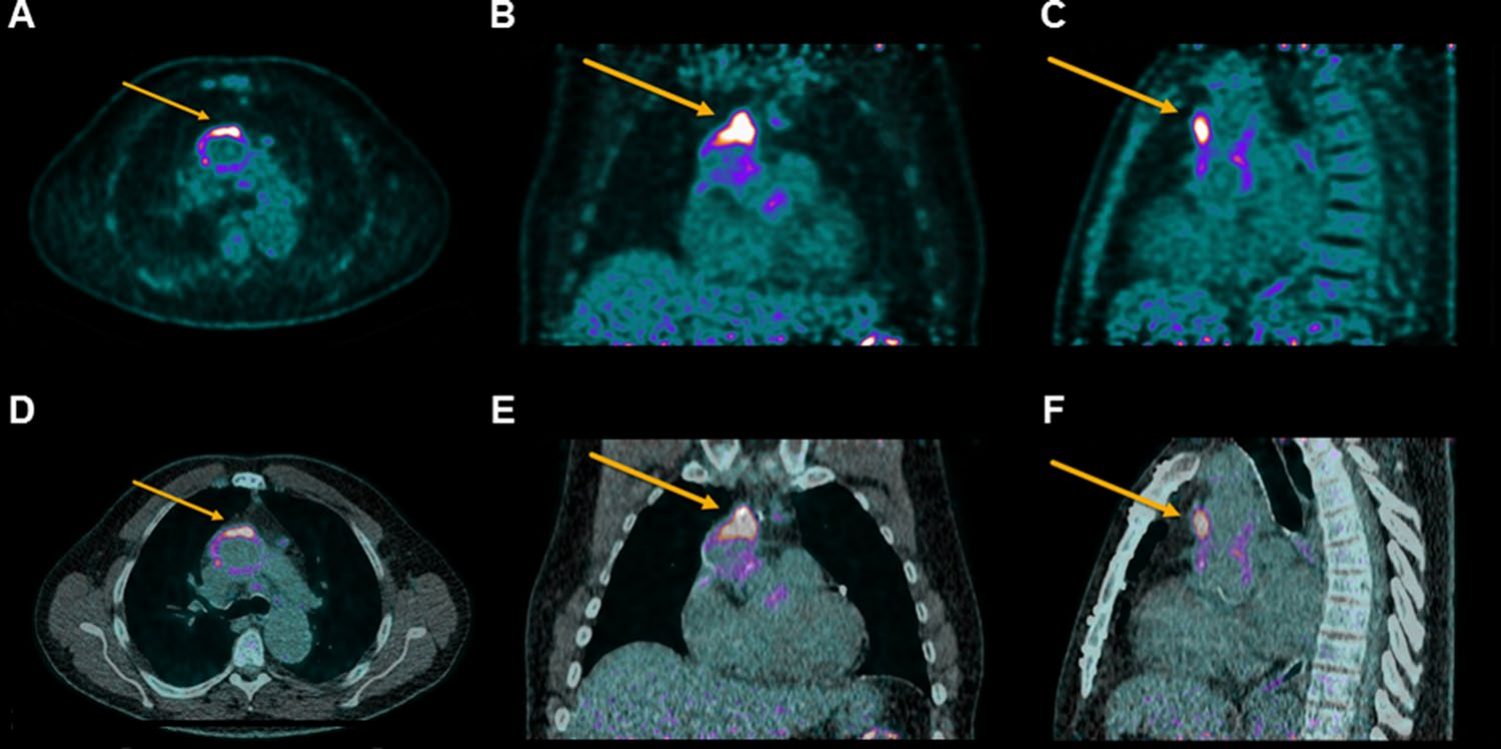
Abnormal, inhomogenous, focal uptake of the ascending aorta on PET only (A-C) and on PET/CT (D-F) in axial (A&D), coronal (B&E) and sagittal (C&F). Lesion uptake parameters were SUV_max_ 18.5, SUV_mean_ 3.0 SUV_peak_ 11.1
